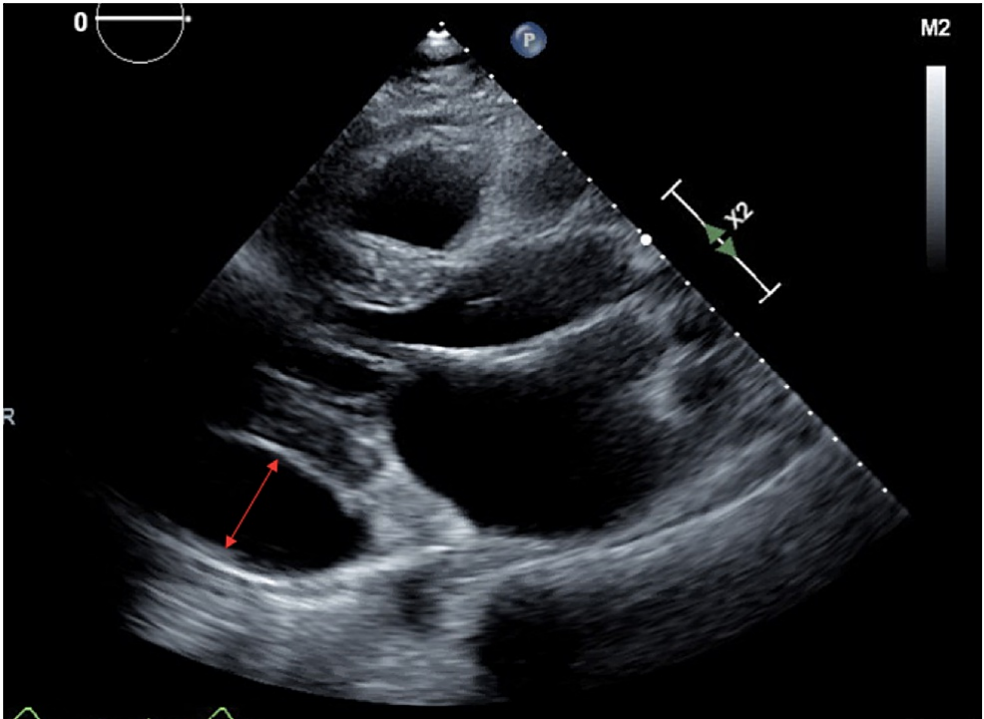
Transthoracic echocardiographic imaging showing moderate-sized posterior pericardial effusion.
